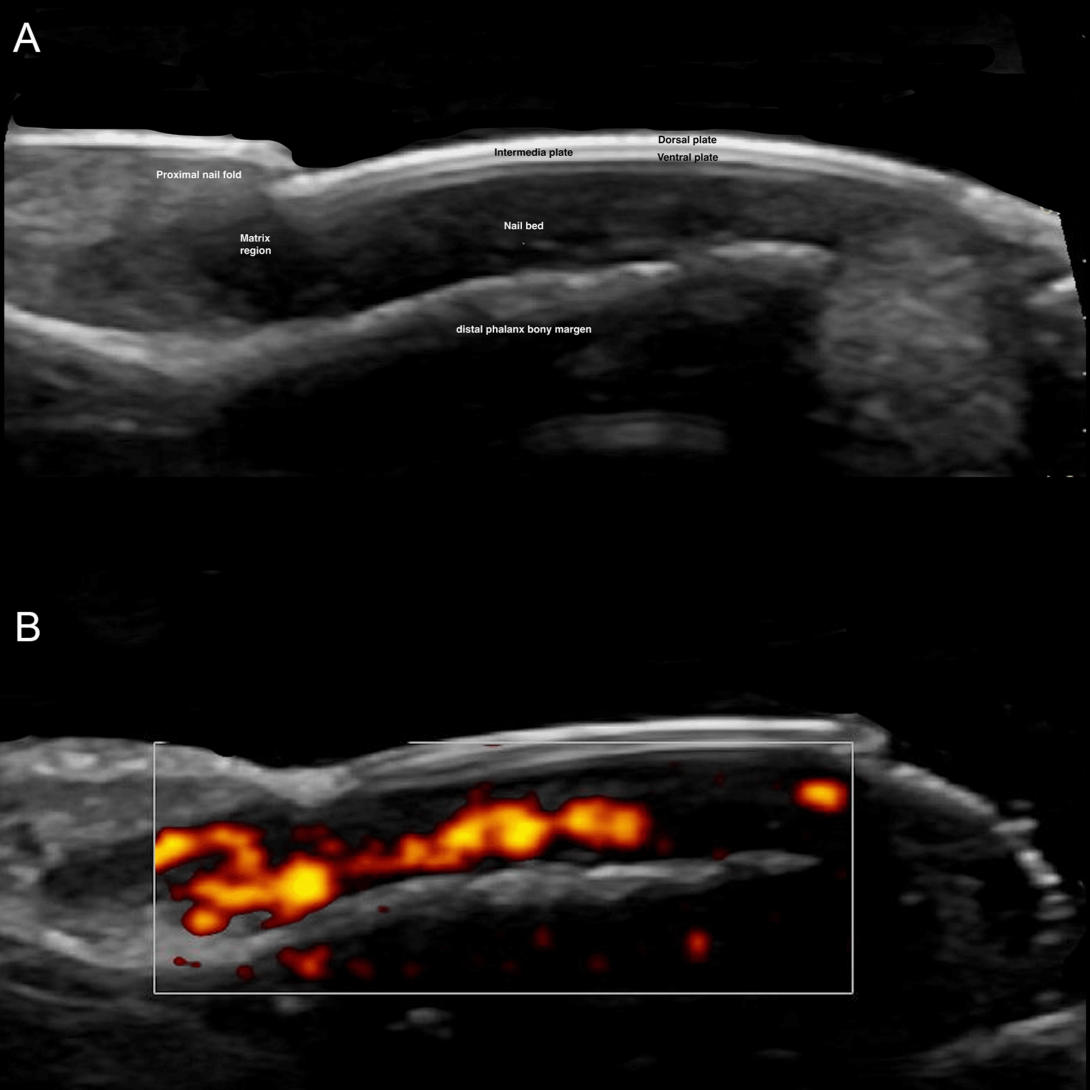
Ultrasound anatomy of the nail unit A: HFUS, a longitudinal greyscale image of the nail unit highlighting all normal anatomical structures with clarity; B: longitudinal Doppler, duplex color ultrasound image of the nail, displaying the normal vascularization. This detailed view highlights the precise flow patterns within the nail unit. Original unpublished image, courtesy of Dr. Claudia Gonzalez. HFUS: high-frequency ultrasound
